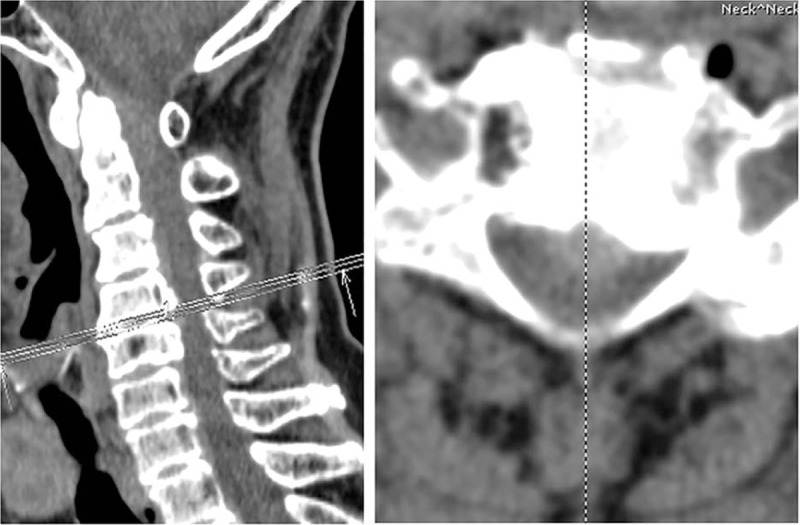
Cervical spinal computed tomography revealed stenosis of spinal canal at the lower cervical.
